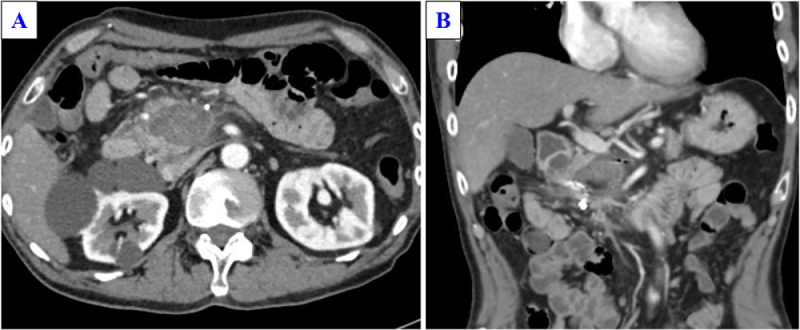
Preoperative computed tomography (CT) findings. (A) Axial and (B) coronal abdominal CT images immediately before surgery showing a 4.0 cm mass in the pancreatic head with features suggestive of thrombosis. Extensive occlusion of the superior mesenteric vein (SMV) was persistent, and the vein was not visualized.
